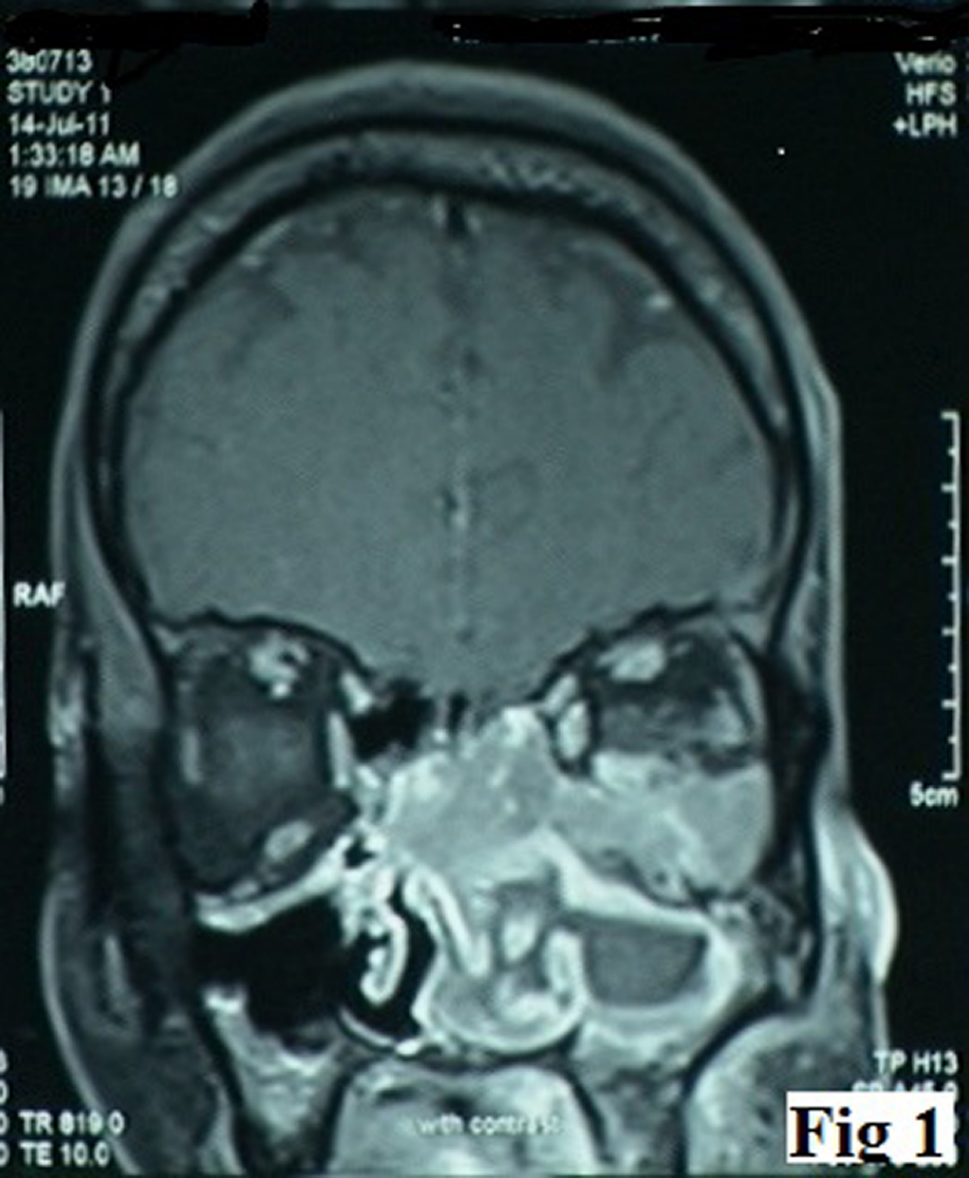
CT scan showing an intranasal mass involving the bilateral ethmoid sinuses with the destruction of the ethmoid septae and the medial wall of the left orbit.
